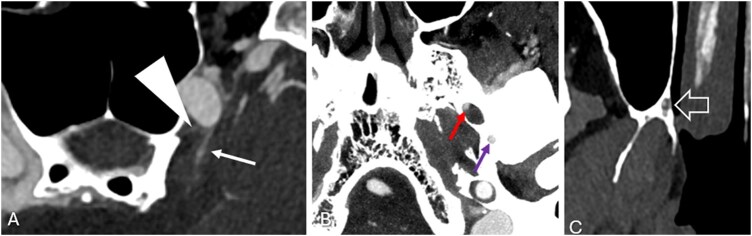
PCD-CTA axial images in (A) show meningohypophyseal trunk branches, including the inferior hypophyseal artery (white arrowhead) and the marginal tentorial artery (Bernasconi–Cassinari artery) supplying the tentorial meninges (white arrow). PCD-CTA in (B and C) shows small branches of the maxillary artery, including the artery of foramen rotundum (red arrow in B), the middle meningeal artery within the foramen spinosum (purple arrow in B), and the greater and lesser palatine arteries (white block arrow in C). Abbreviations: CTA, CT angiography; PCD, photon-counting detector.
